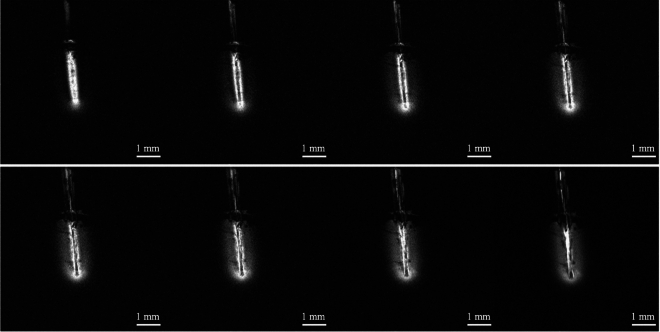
In vivo MRI of CED of contrast agent solution. Series of T1-weighted FLASH MRI images acquired with the implanted microcoil as a receiver. FLASH sequence was used with the following parameters: slice thickness = 0.4 mm, FOV = 10 × 10 mm^2^, in-plane resolution = 26 × 26 μm^2^, acquisition time = 8 min 19 s. The contrast agent solution was infused at a flow rate of 0.1 μL/min. The delay between each acquired image was 16 min. The first image, top row on the left was acquired before the start of the CED of the solution.
